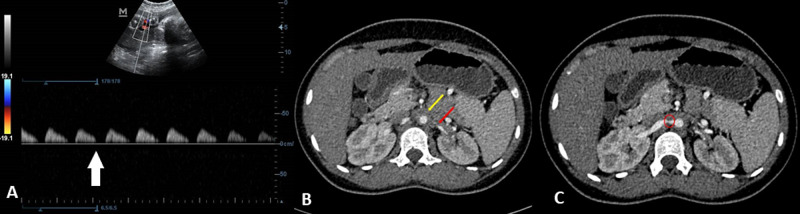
(A) Absent end-diastolic flow (white arrow) in the fetal umbilical artery on color Doppler ultrasound. (B) CECT arterial-phase image (axial section) showing narrowing of the maternal abdominal aortic lumen with hypodense wall thickening (yellow arrow) and severe narrowing of the left renal artery (red arrow). (C) CECT arterial-phase image of the maternal abdomen (axial section) showing severe focal stenosis of the proximal right renal artery (red circle). CECT, contrast-enhanced computed tomography
